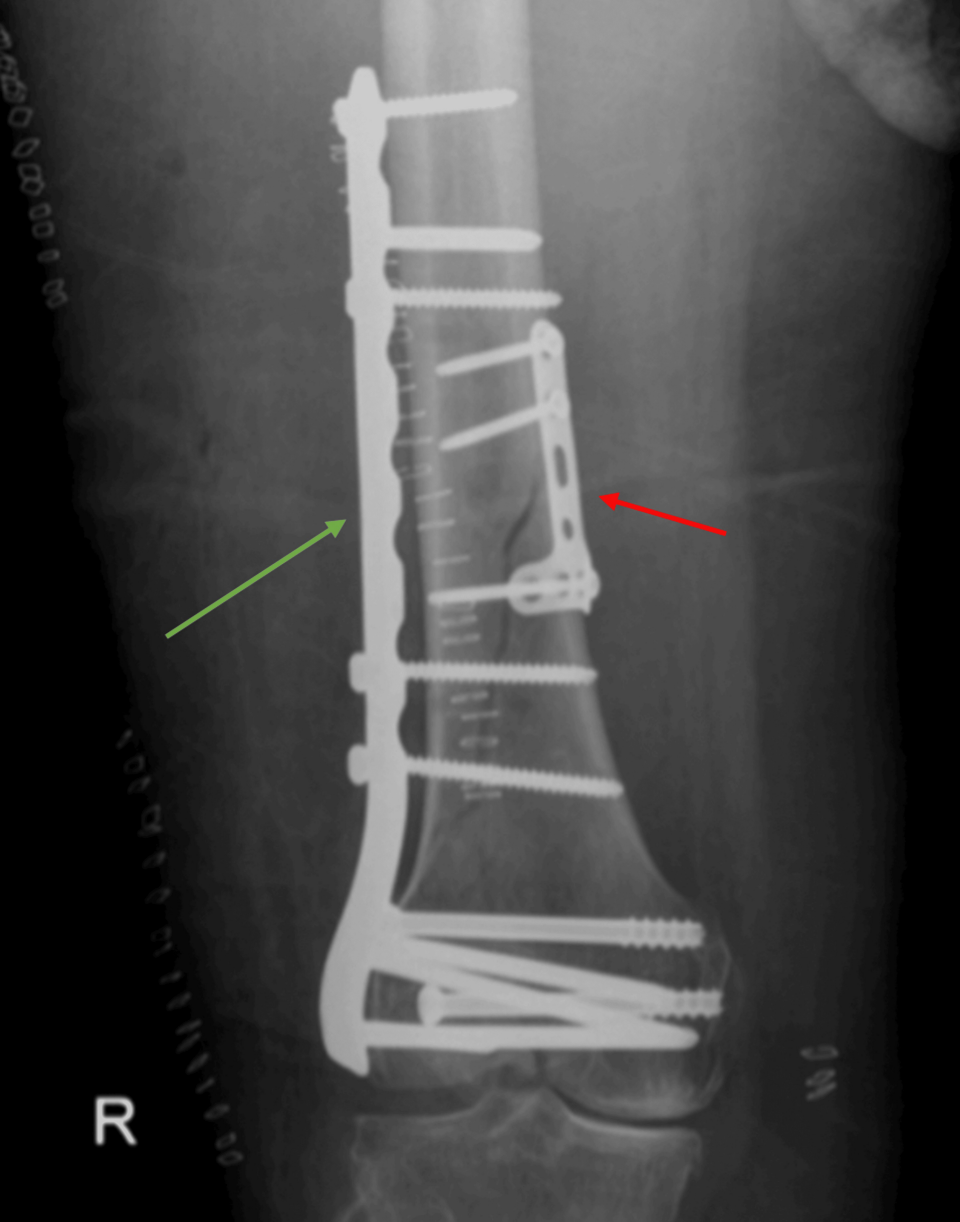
The immediate postoperative X-ray The postoperative X-ray displays an anterior-posterior (AP) view of the right femur. The T-plate has two screws inserted proximally and one screw placed distally, as indicated by the red arrow. The green arrow shows a lateral locking plate. The fixation resulted in a significant reduction of the fracture fragments.
